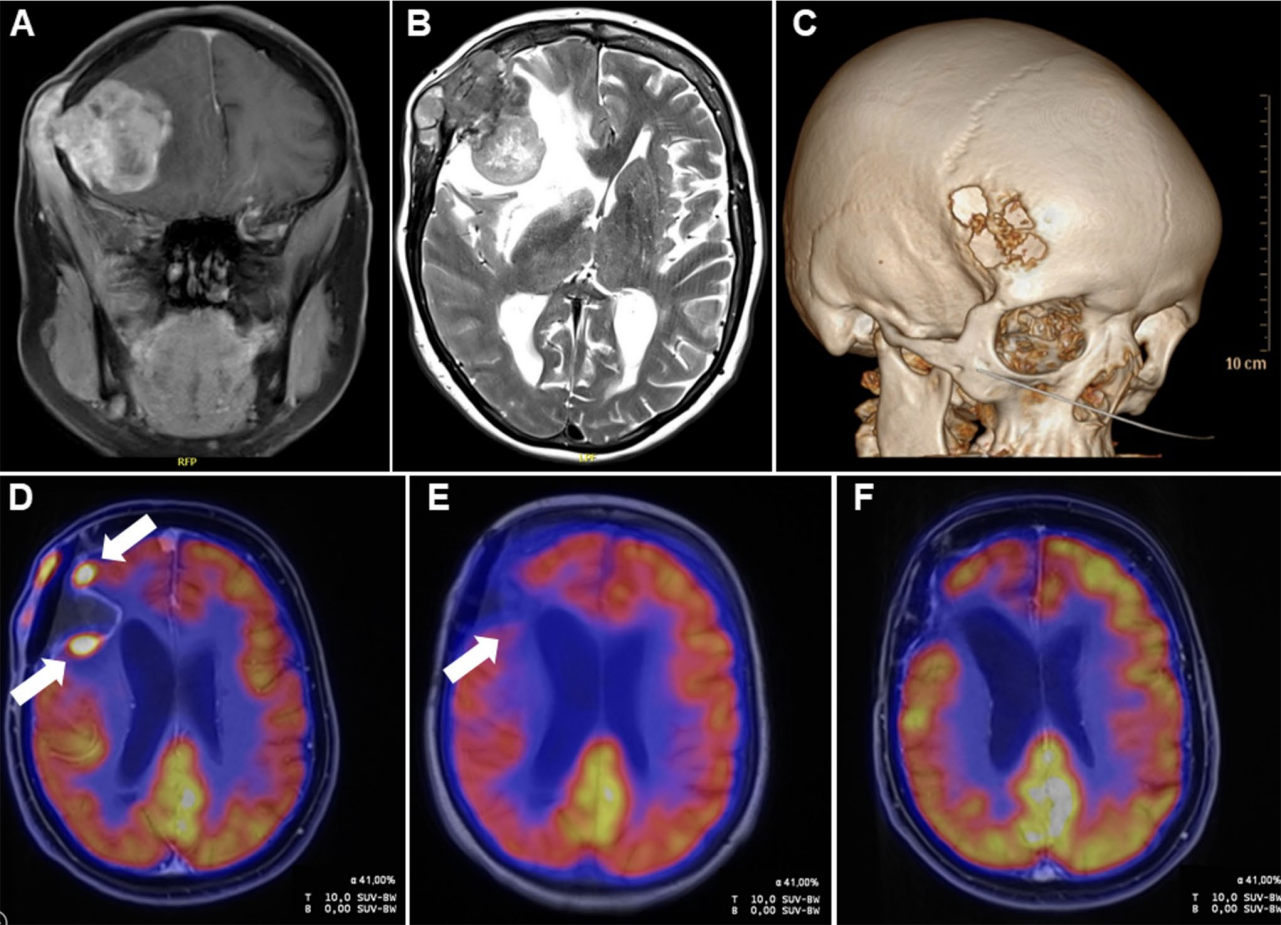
Imaging of the osteoblastic osteosarcoma. MR-imaging of the osteoblastic osteosarcoma at first diagnosis on coronal T1-weighted-flair (A) and axial T2-weighted-TSE imaging (B). Osteoblastic osteosarcoma visualized by 3D CT-scan (C). Early postoperative 18 F-FDG PET/MRI revealed locoregional recurrence (D, arrows). In 18 F-FDG-PET/MRI after three cycles of Euro-B.O.S.S. residual vital tumor on the margins of the resection cavity could not be ruled out (E, arrow). No signs of residual tumor in the PET/MRI after 22 months of palbociclib therapy (F)
